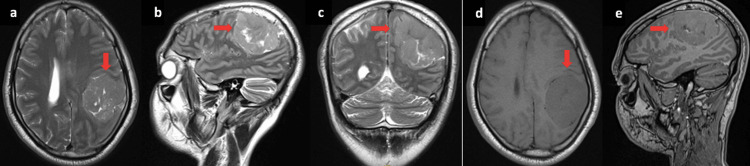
Cerebral MRI: (a) T2 axial, (b) T2 sagital, (c) T2 coronal, (d) T1 axial, (e) T1 sagital
